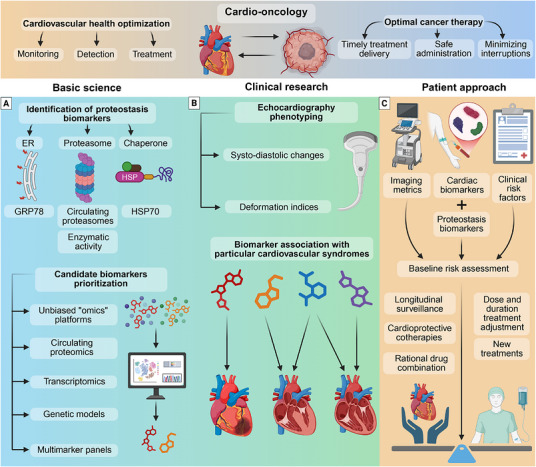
Comprehensive view of cardio‐oncology from research to clinical application. (A) Basic research is crucial for identifying new biomarkers and prioritizing them using various methods. (B) Combining echocardiographic parameters with biomarkers linked to specific cardiovascular conditions helps identify phenotypes that are prone to cardiotoxicity. (C) These findings facilitate a personalized, patient‐focused approach by accurately assessing the baseline cardiac risk using imaging metrics, biomarkers, and clinical data, aiding physicians in choosing customized anticancer treatments. Similarly, ongoing cardiac monitoring during anticancer therapy using these tools may optimize treatment plans through dose modifications, drug combinations, and cardioprotective co‐therapies, ultimately preserving cardiac function and treatment effectiveness. Created with BioRender.com.
